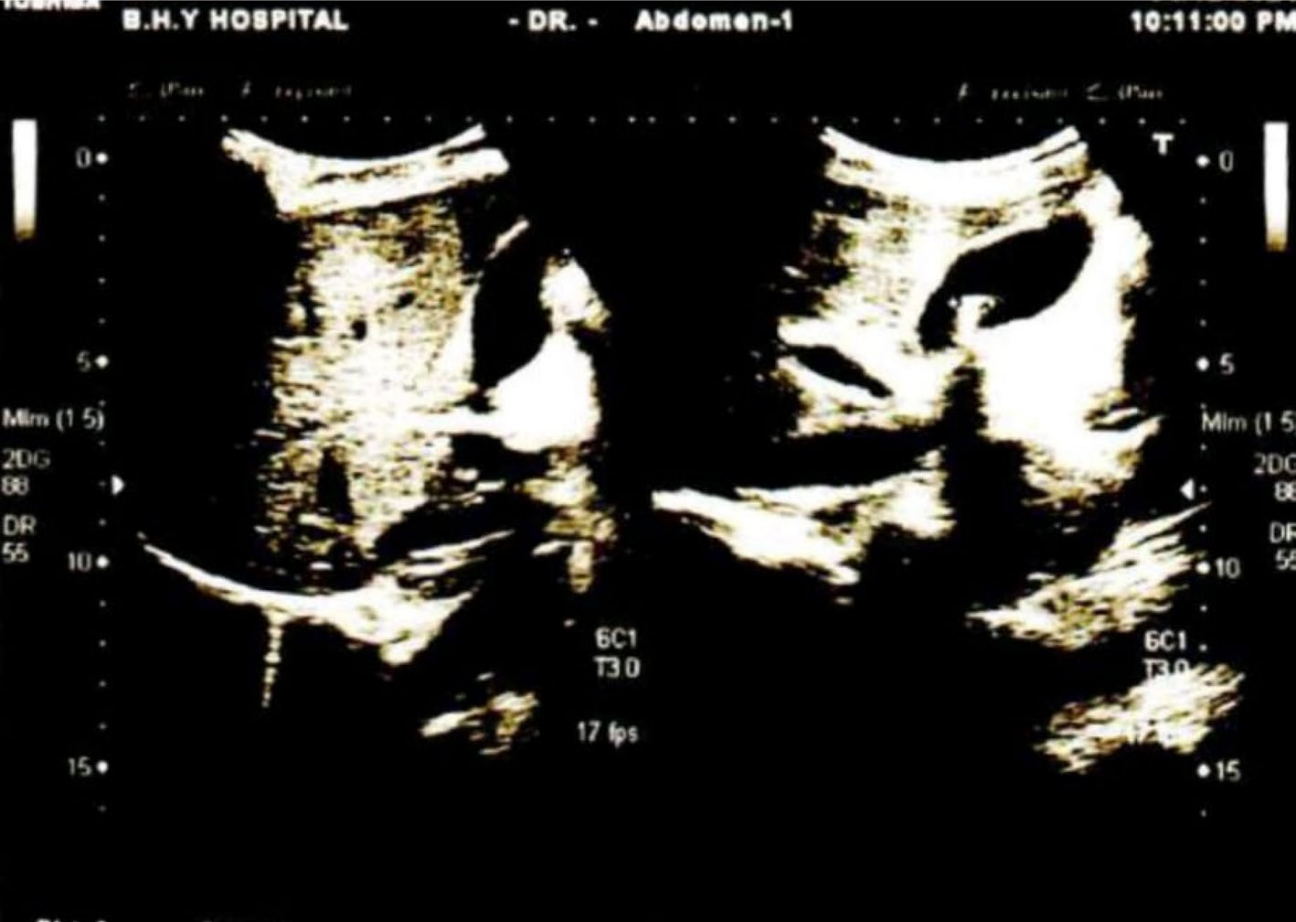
Abdominal ultrasonography showing the gallbladder in longitudinal view with multiple echogenic intraluminal calculi producing posterior acoustic shadowing. The adjacent liver appears normal in echotexture, with no sonographic evidence of biliary ductal dilatation.
